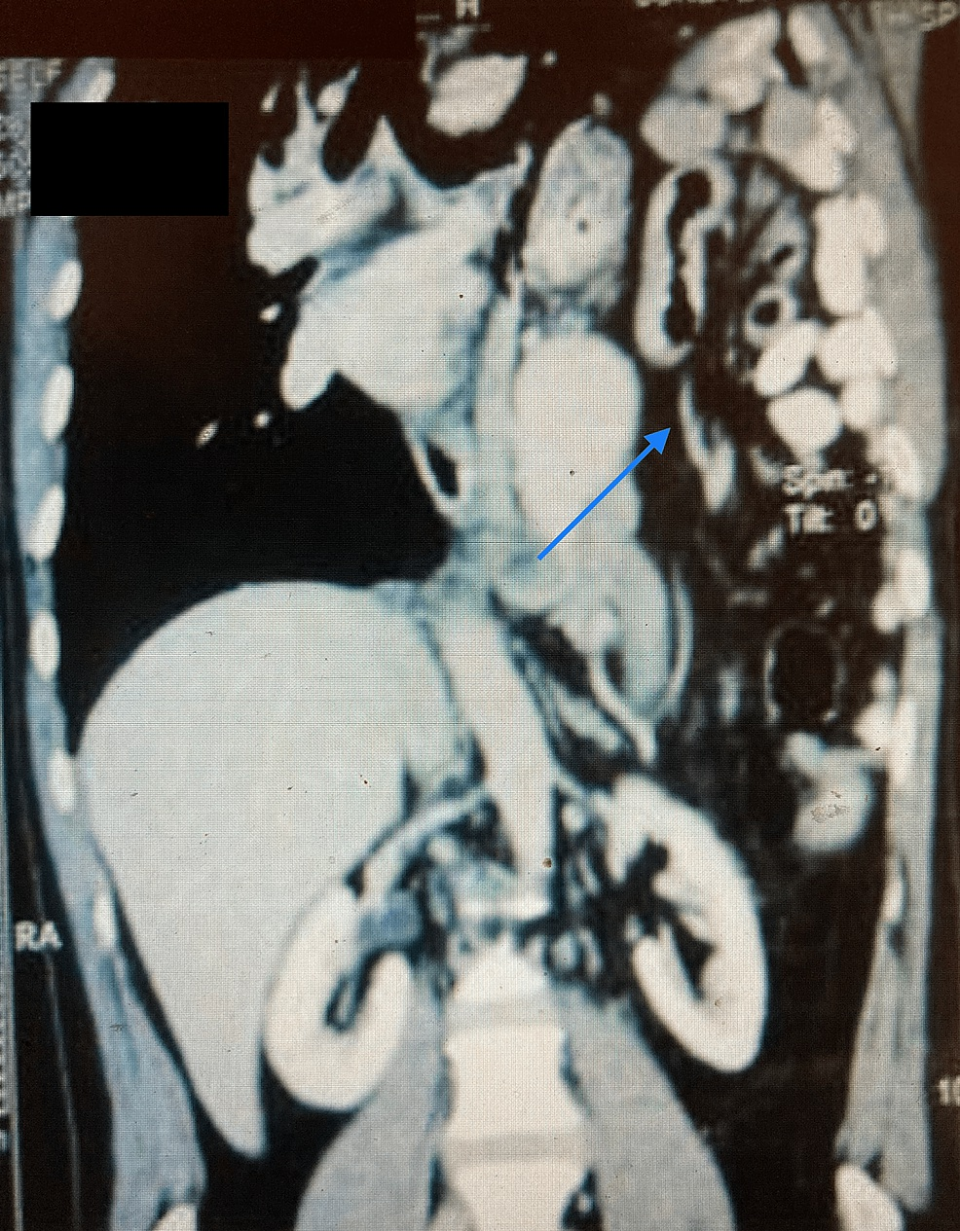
Contrast-enhanced computed tomography of the abdomen and chest showing a large diaphragmatic hernia with herniated bowel loops in the transverse colon, the splenic flexure of the colon with mesentery, the spleen, and the tail of the pancreas, as well as the collapsed left lung.
